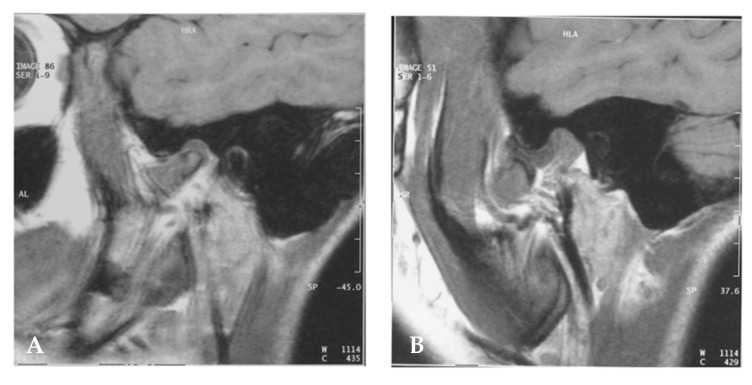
MRI of the right TMJ shows a meniscus which, although thin, is normally positioned between the bony heads with both open mouth (A) and closed mouth (B). In the closed-mouth study, anterior dislocation of the meniscus is documented with subsequent recapture of the same in the open-mouth study, a finding compatible with a condylar-meniscal incoordination syndrome. The MRI examination also documented a modest hypotrophy of masticatory muscles on the right.
